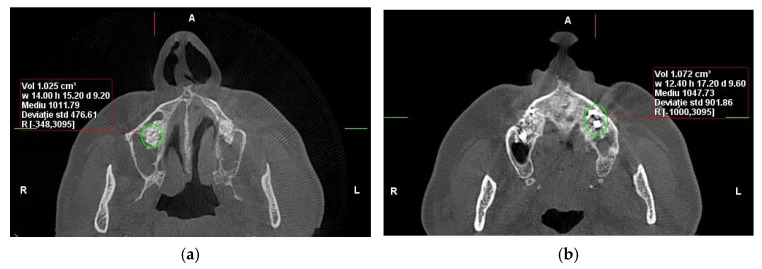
CBCT reconstructions in the axial plane were used to assess bone density (HU) in the area of sinus augmentation at the 10-year follow-up: (a) with RS administration, (b) without adjuvant therapy involving RS (Personal Collection).
